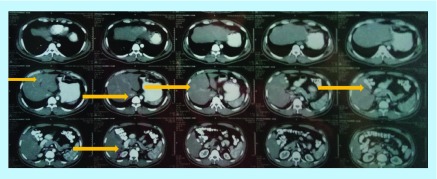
CT scan performed 24 months postsurgery displays multiple hypodense lesions in liver.
